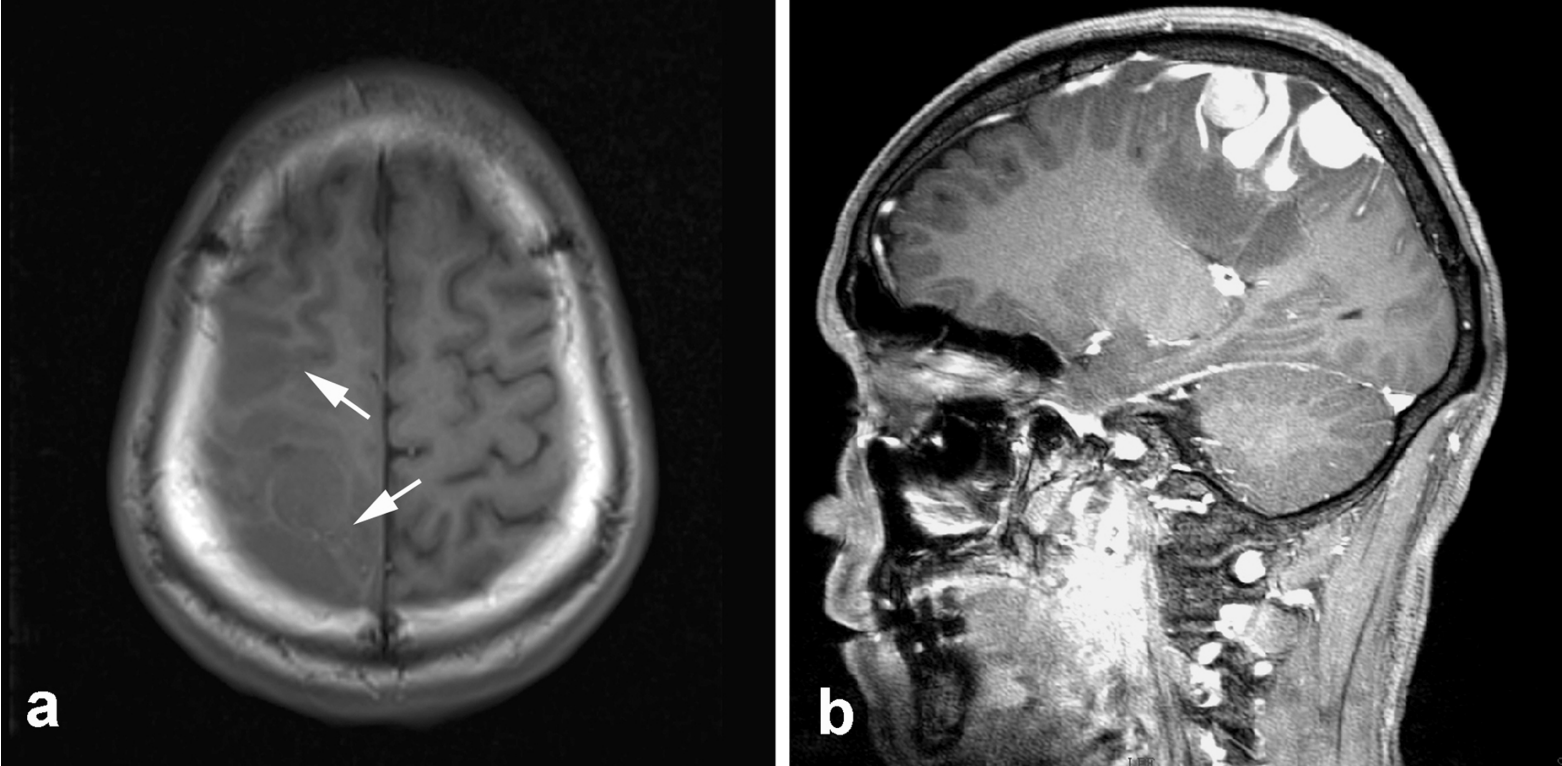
Neuroimaging findings of lesion. (a) Preoperative axial T-1 weighted magnetic resonance imaging (MRI) revealed multifocal hypointense lesion localized in right frontoparietal lobe (white arrowed). (b) Sagittal MRI shows the well-circumscribed enhanced lesion extending from the superficial cortex along the meninges into the depth of white matter.
